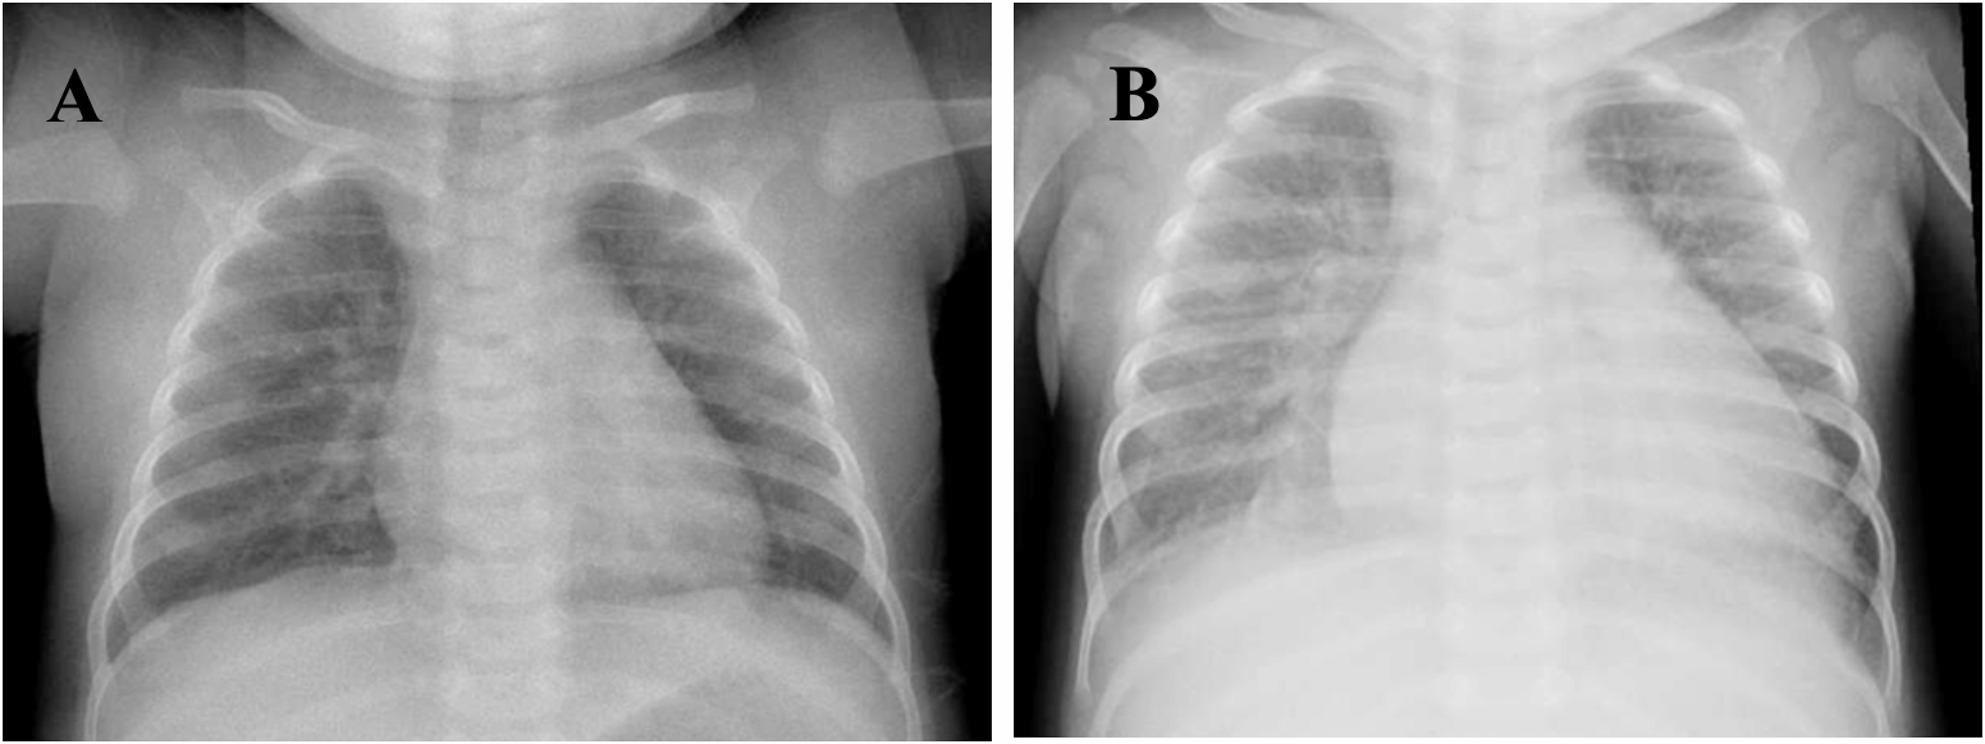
Serial chest radiographs demonstrating progression to severe cardiomegaly. A Chest radiograph obtained at four months of age during initial hospitalization demonstrating a normal cardiac silhouette. B Chest radiograph obtained at the final presentation at six months of age demonstrating marked cardiomegaly (cardiothoracic ratio 0.69) with pulmonary edema
